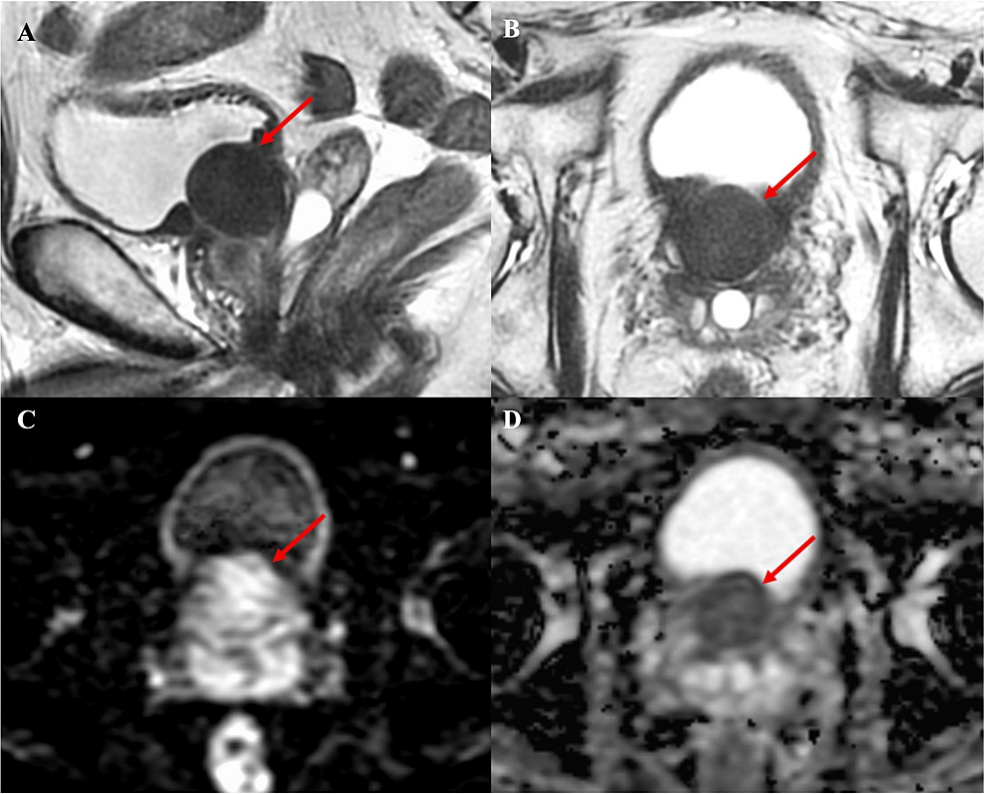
MRI of the prostate T2-weighted axial (A) and sagittal (B) images show a mass with a clear border and low signal intensity. (C, D) Diffusion weighted imaging (DWI) and apparent diffusion coefficient (ADC) mapping show diffusion restriction.
